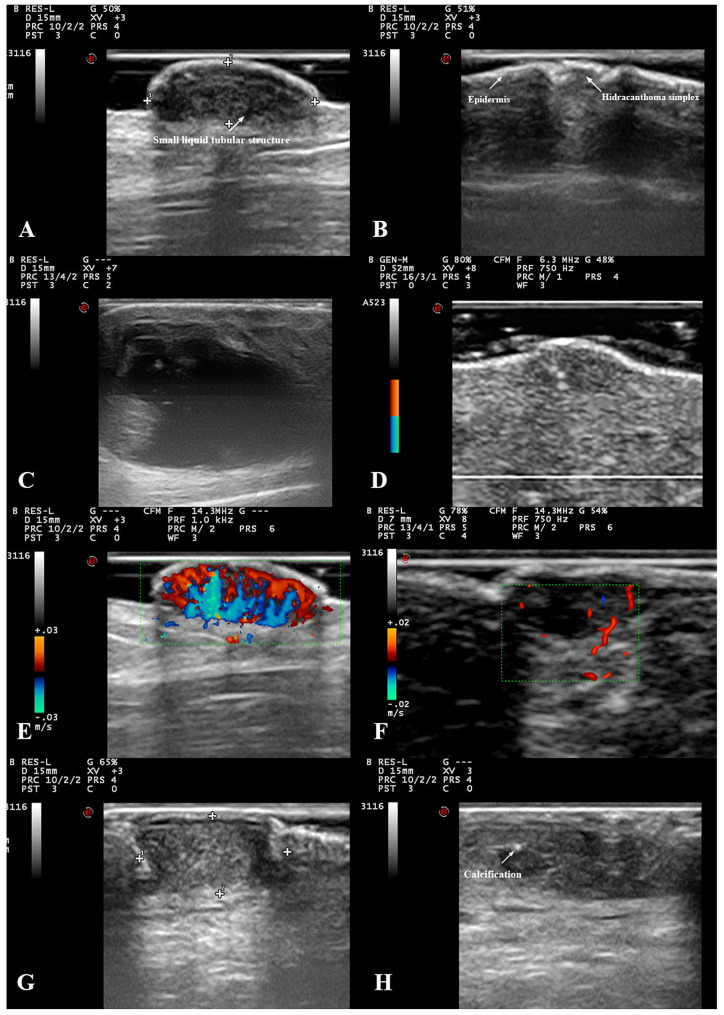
The VHF ultrasound characteristics of 24 poromas. A: eccrine poroma involving the epidermis and dermis layers, with heterogeneous hypoechoic and small liquid tubular structure; B: hidracanthoma simplex only involving the epidermis; C: poroid hidradenoma with liquid-based mixed echo; D: dermal duct tumor; E: grade Ⅲ of blood flow with Adler’s method, dendritic blood vessels; F: grade Ⅱ of blood flow; G: enhanced rear echo; H: calcification
